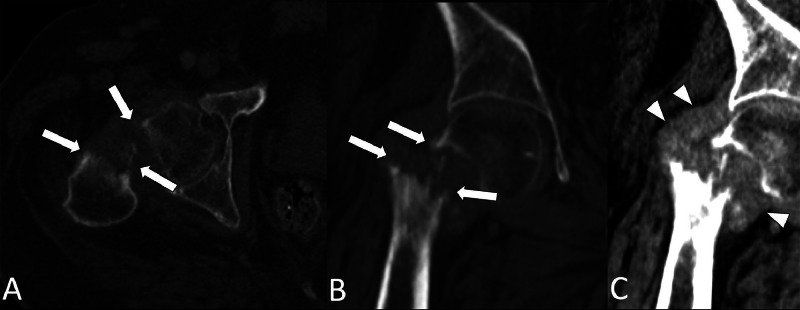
Axial and coronal CT images (A-B) of the pelvis shows a pathologic fracture of the right hip (white arrows). Coronal view (C) shows a surrounding soft tissue mass (arrowheads).
